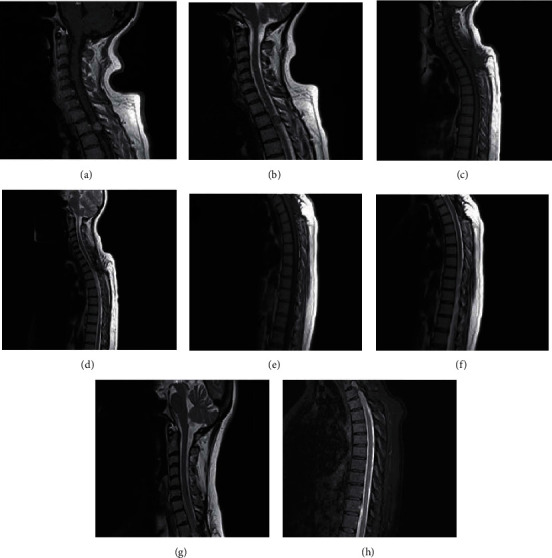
MRI images on preoperative, 3 months postoperative, 1 year postoperative, and current period. (a, b) (08.12.2017) Preoperative MRI reveals a lesion 12 × 10 mm in diameter, hypointense in both T1-w and T2-w, heterogeneously contrast enhancing with gadolinium. (c, d) (06.03.2018) Postoperative MRI reveals a paracentral mass located in the posterior edge of the spinal canal with minimal contrast enhancement. (e, f) (19.01.2019) Control MRI reveals. (g, h) (18.02.2021): MRI at current presentation reveals a lesion which is moderately hypointense in T1-w and hypointense in T2-w with homogeneous contrast enhancement.
